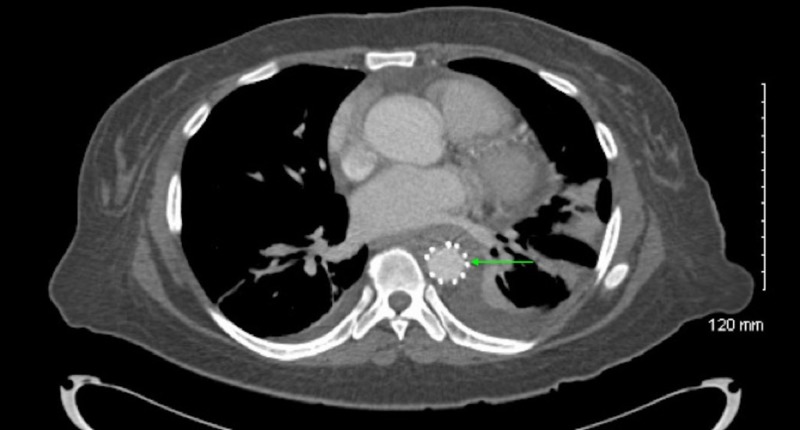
CT scan of chest post-procedure Green arrow showing TEVAR aortic repair CT: computed tomography; TEVAR: thoracic endovascular aortic repair
